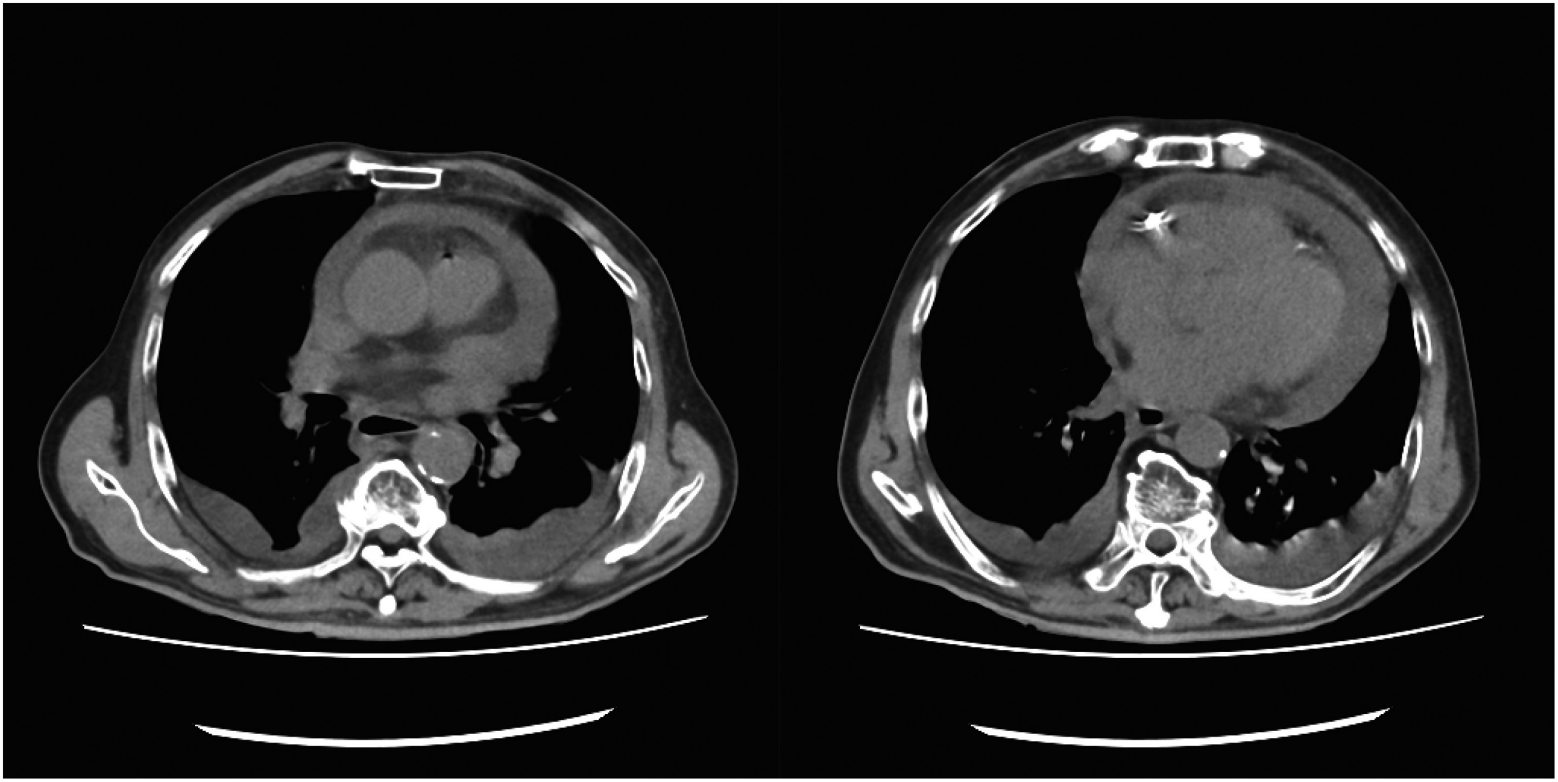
Preoperative computed tomography showing pericardial effusion. There is no evidence of dissection in the ascending aorta.
